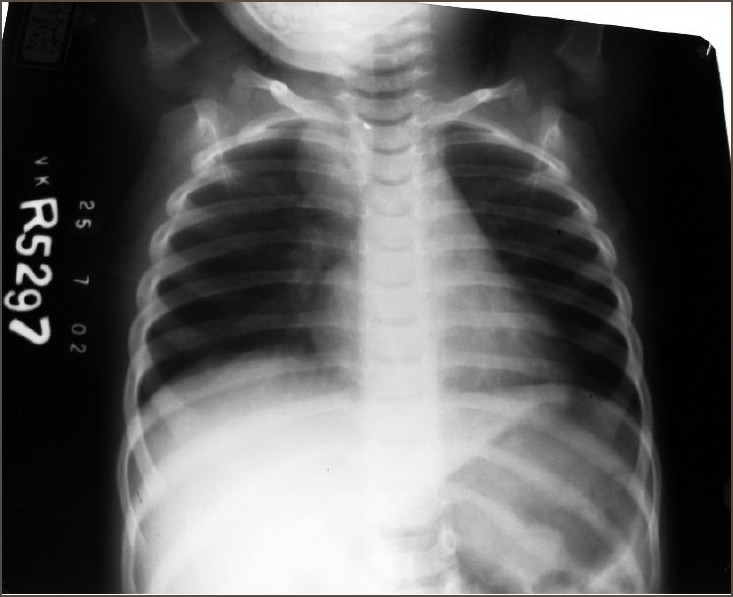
An X-ray chest showing a right paratracheal soft tissue density
